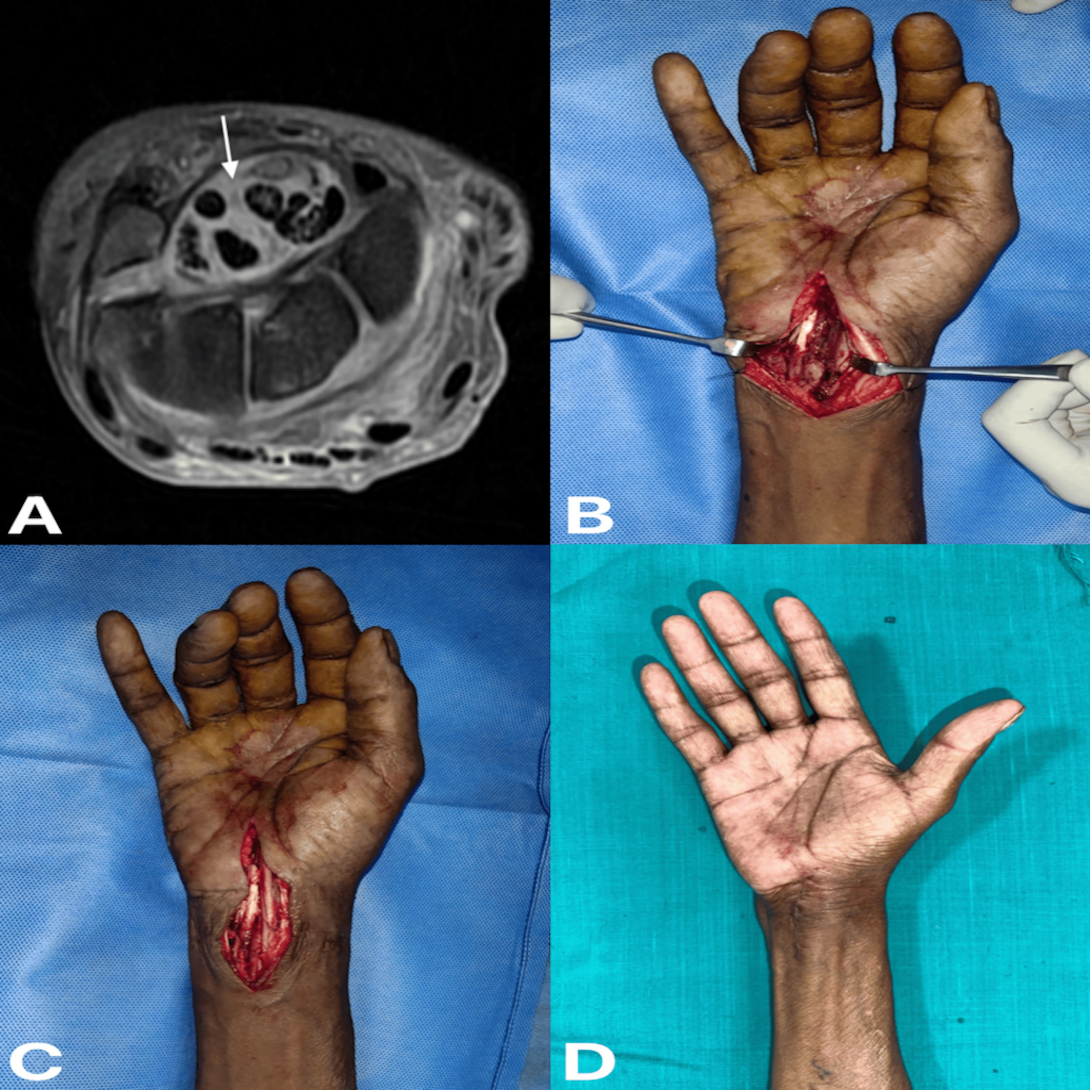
(A) Magnetic resonance imaging of right wrist showing axial section, (B) intra-operative picture showing infected synovium, (C) intra-operative picture showing median nerve decompression, and (D) six months post-operative clinical picture of right wrist (A) Arrow denotes synovial thickening and edema around the flexor tendon.
